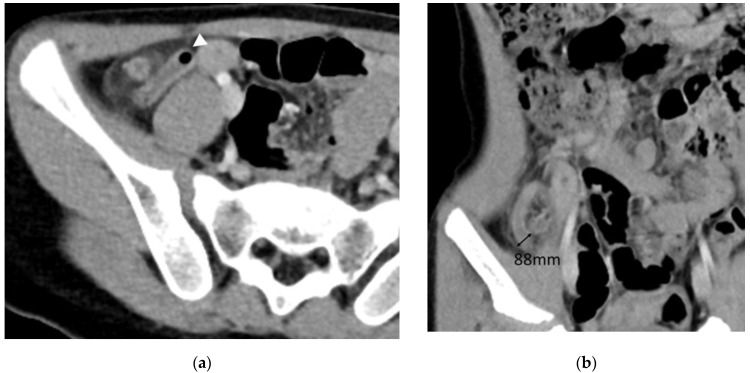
A 6-year-old male with pathologically confirmed complicated appendicitis. (a) Axial and (b) coronal CT images reveal an 88 mm maximum appendiceal diameter and intraluminal air density (arrowhead). The laboratory test showed increased level of WBC (16.01 × 10^3^/uL).
